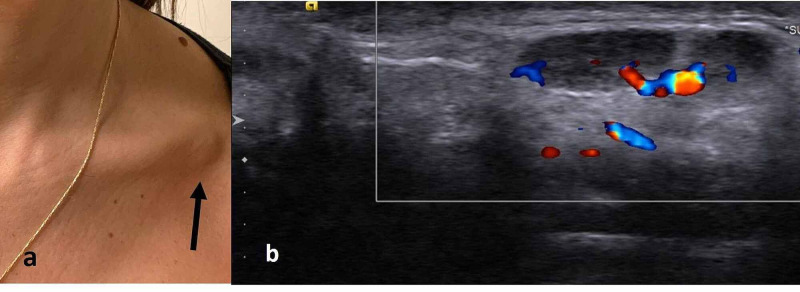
(a) Focal swelling in the left infraclavicular area that prompted the study (arrow). (b) Ultrasound image of the area demonstrating enlarged lymph nodes with a slightly hypoechoic cortical layer but with preserved hilum and blood supply.
